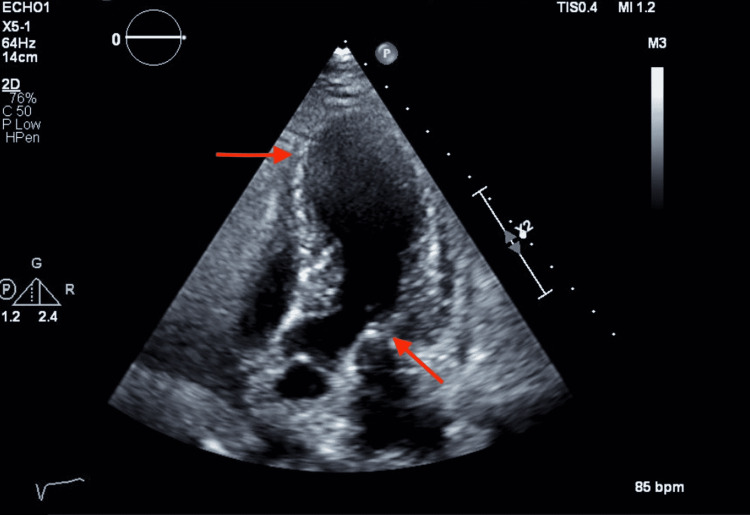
Echocardiography demonstrating the left ventricle with a hypokinetic apex with ballooning as denoted by the top arrow. Basal segments contract toward each other, as denoted by the bottom arrow. These forms the typical “octopus-pot” appearance.
